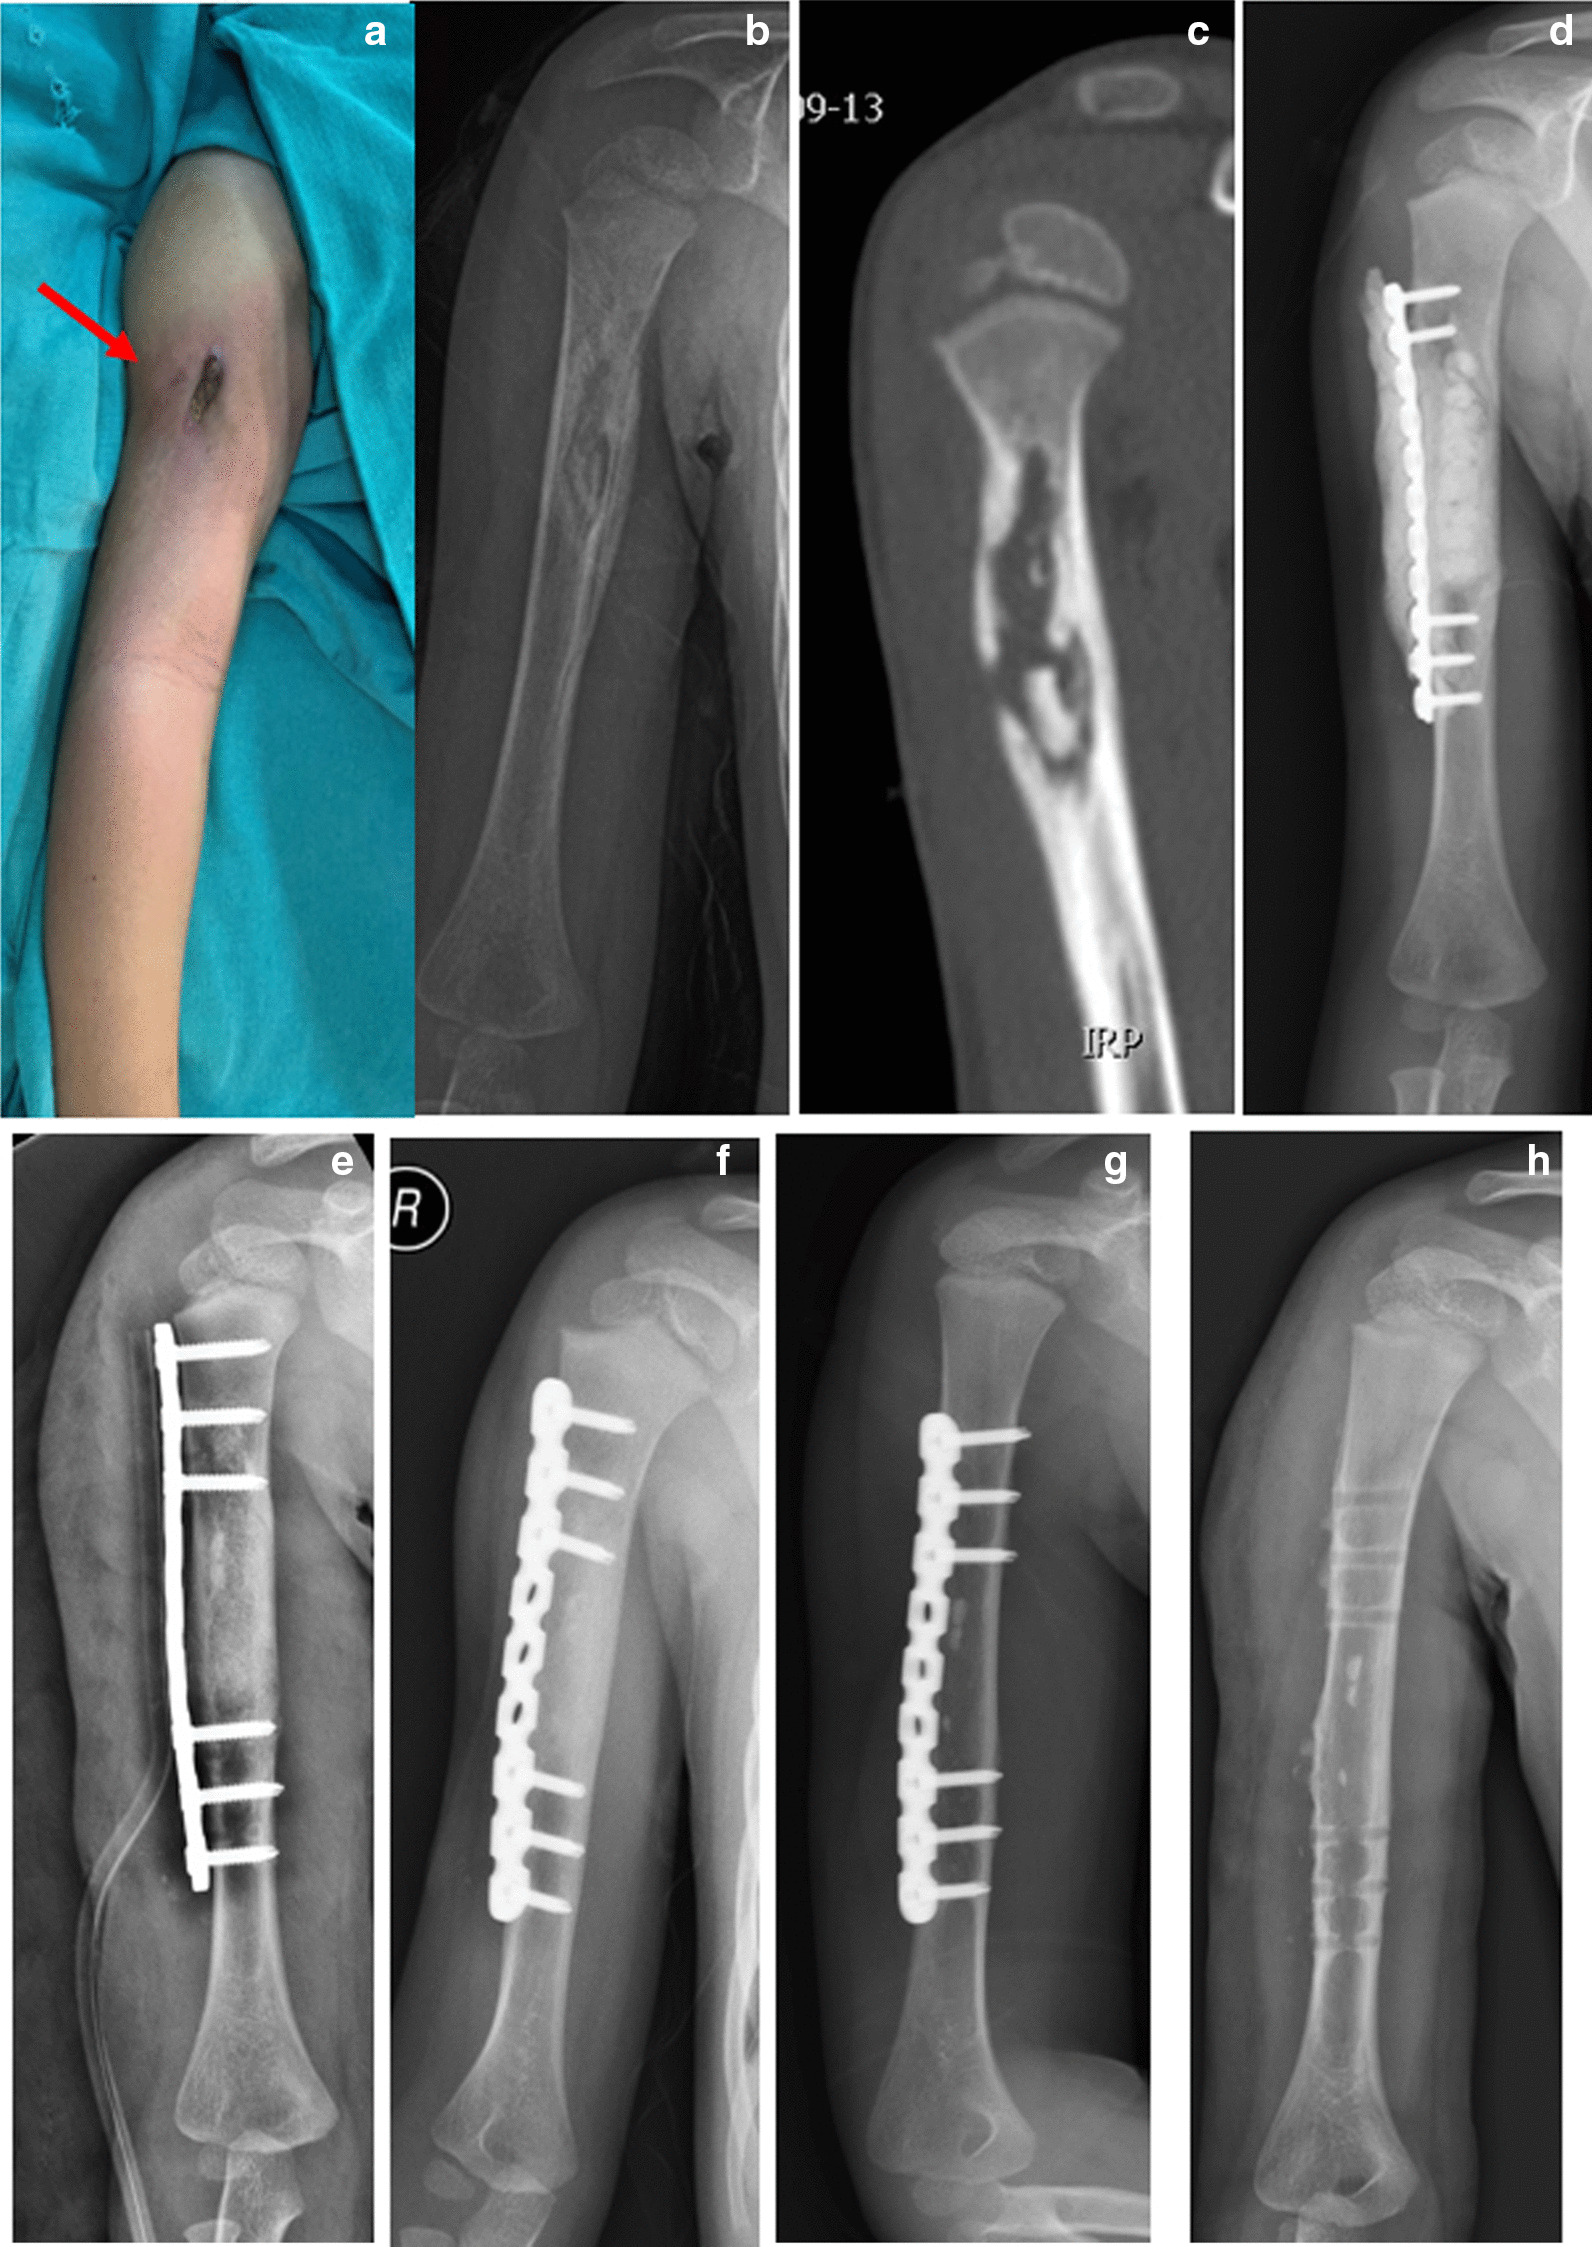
Case 5: a 3-year-old boy who has recently presented with recurrent swelling and drainage from his right upper arm. At the age of 2, he underwent surgery due to swelling, redness, and pain with no preceding trauma. Afterward, a sinus tract formed at the incision (red arrow). a Pre-operative presentation of an affected limb. b Anteroposterior (AP) radiograph shows chronic osteomyelitis of the right humerus. c CT shows a sequestrum. d In the first stage of surgery, debridement and locking plate were performed and antibiotic-loaded bone cement was used to fill the bone defect area. e AP radiograph after bone graft in the second stage of surgery. f AP radiograph shows bone defect healed at 3 months after bone grafting. g AP radiograph shows bone defect remodelled at 26 months after bone grafting. h AP radiograph shows that the internal fixation was removed at 26 months after bone grafting
